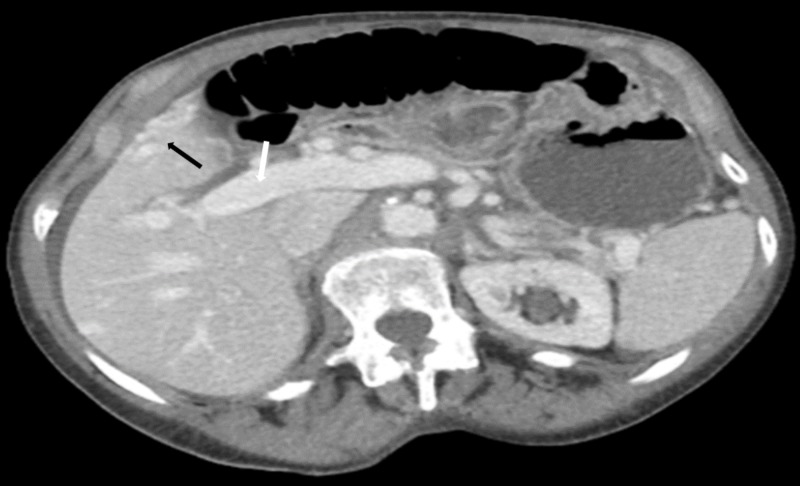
Cross-sectional view of CT scan of the abdomen and pelvis Cross-sectional view showing dilated portal vein measuring up to 1.8 cm at the porta-hepatis (white arrow) and multiple dilated vascular channels (black arrow) in the right hepatic lobe (segment V). An intrahepatic portosystemic shunt between the segment V portal venous branches and a peripheral hepatic vein draining into the middle hepatic vein was visualized (not shown here).
